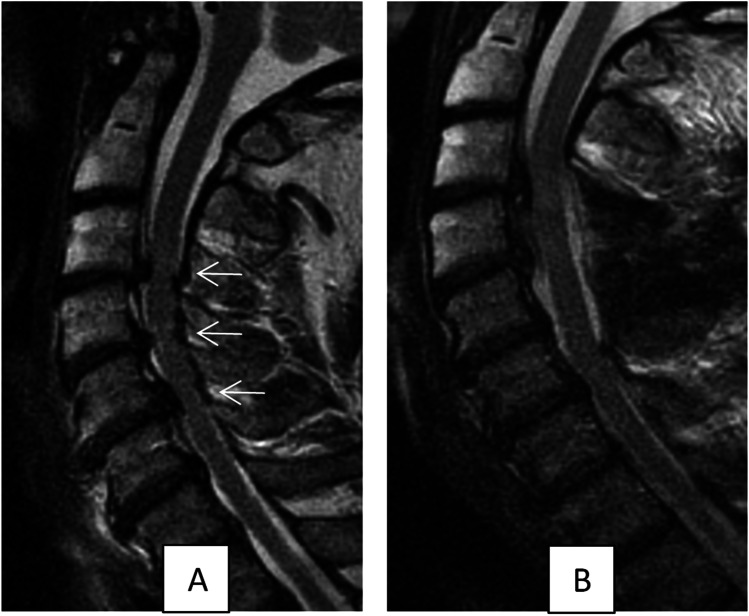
A Preoperative sagittal MRI (T2-weightened) showing spinal stenoses at segments C 3/4, C 4/5 and C 5/6 (arrows). B Postoperative MRI showing status after laminectomy C 4 and C 5 and dorsal shift of the spinal cord
